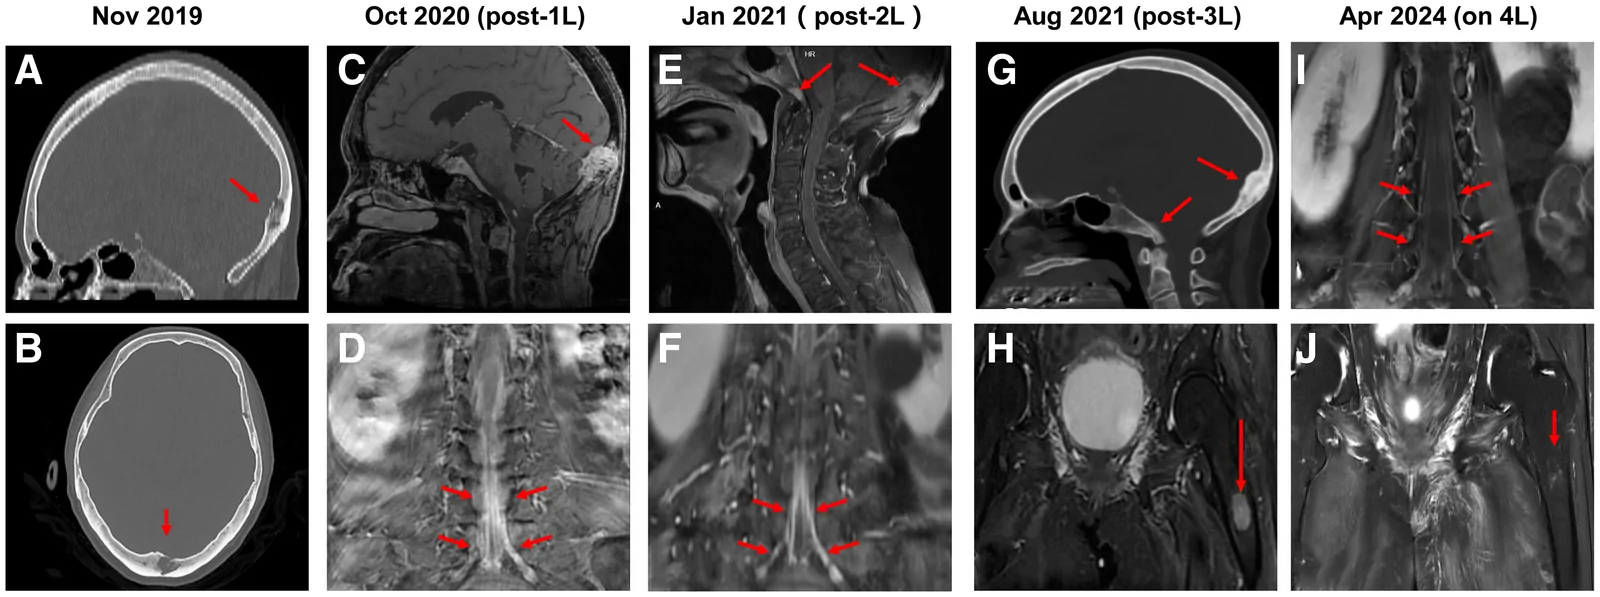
Key radiological findings during the clinical course. (A, B) Sagittal (A) and axial (B) head CT (bone window) in November 2019 showing the initially detected occipital bone metastasis. (C, D) Sagittal cranial MRI in October 2020 confirming the occipital lesion (C) and coronal lumbosacral enhanced MRI revealing a new leptomeningeal metastasis involving the cauda equina (D). (E, F) Sagittal cervical enhanced MRI in January 2021 showing a new clival lesion with the occipital metastasis (E) and coronal lumbosacral enhanced MRI demonstrating progression of leptomeningeal disease (F). (G, H) Sagittal head CT (bone window) in August 2021 showing osteoblastic changes with partial relief of occipital/clival lesions (G) and coronal sacroiliac MRI showing a new proximal left femoral metastasis (2.3 × 1.3 cm) (H). (I, J) Coronal spinal and femoral enhanced MRI in April 2024 showing sustained regression of leptomeningeal metastasis (I) and resolution of the femoral lesion (J). Red arrows indicate the metastatic lesions. CT = computed tomography, MRI = magnetic resonance imaging.
